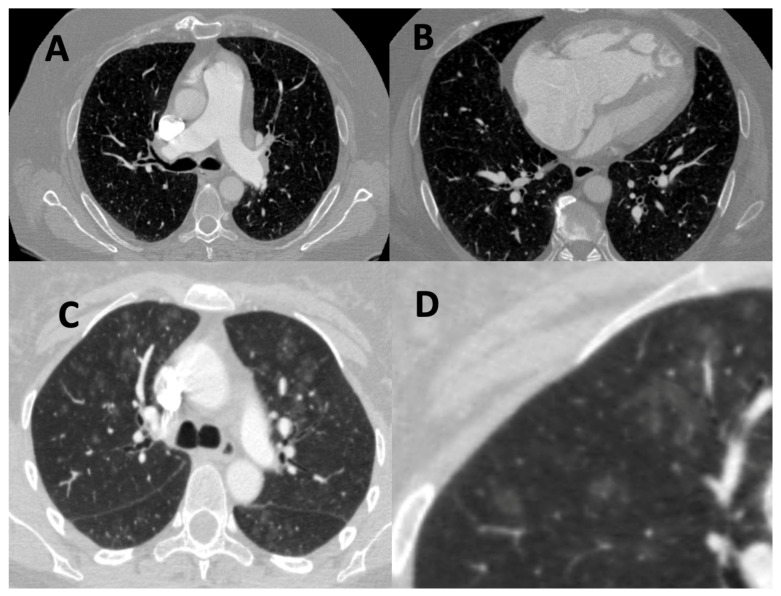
Computed tomography (CT) features of pulmonary arterial hypertension (PAH) on CT. (A) Dilated main pulmonary artery. (B) Right atrial and ventricular dilation with moderate right ventricular hypertrophy and flattening of the interventricular septum. (C) Centrilobular ground glass nodularity. These are a feature of PAH but are also more commonly seen in another sub-phenotypes of pulmonary hypertension, such as pulmonary vascular obstructive disease (PVOD). In PVOD, they are often accompanied by interlobular septal thickening and mediastinal lymphadenopathy. (D) Zoomed in view of regions of centrilobular ground glass nodularity.
